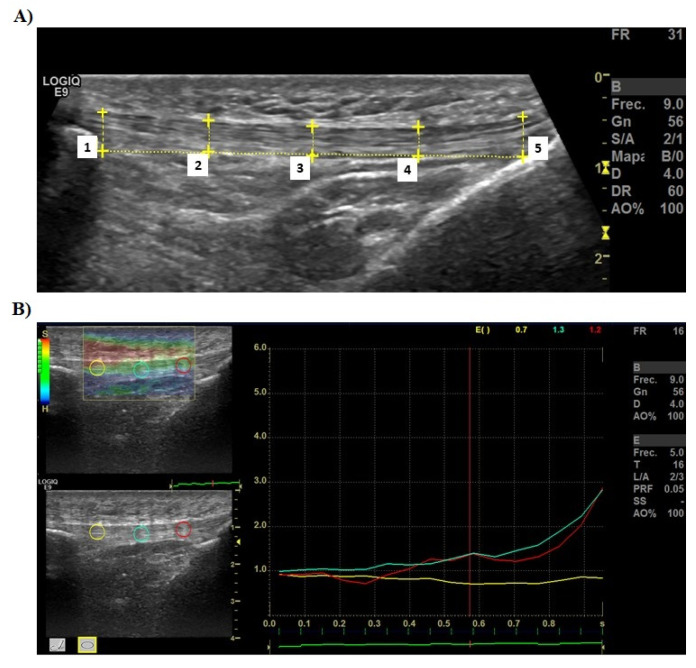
(A) B-mode ultrasound image of the Achilles tendon in a longitudinal plane. (B) Example of elastography measurement of the Achilles tendon.
